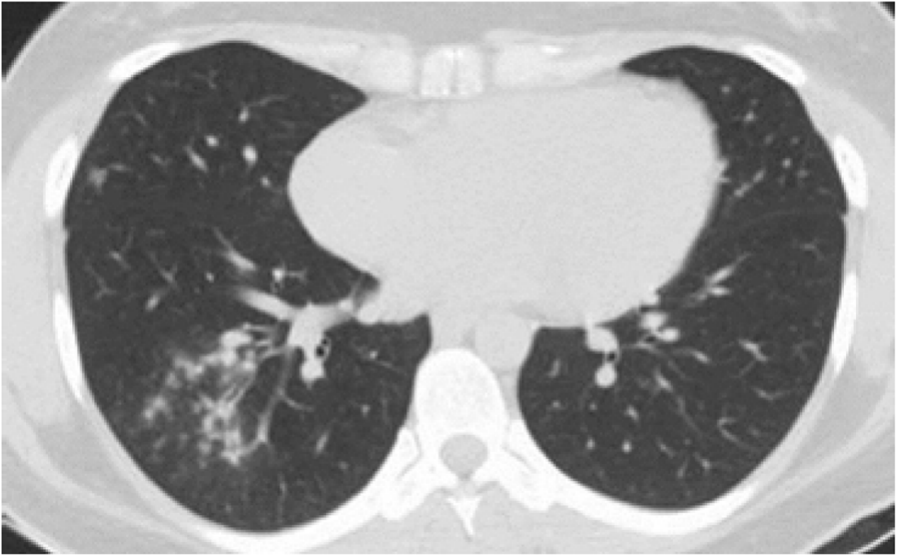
COVID-19 pneumonia: thin section CT shows peribronchial thickening and centrilobular nodules with tree in bud appearance. despite atypical findings for COVID-19 pneumonia, RT-PCR test was positive for COVID-19
